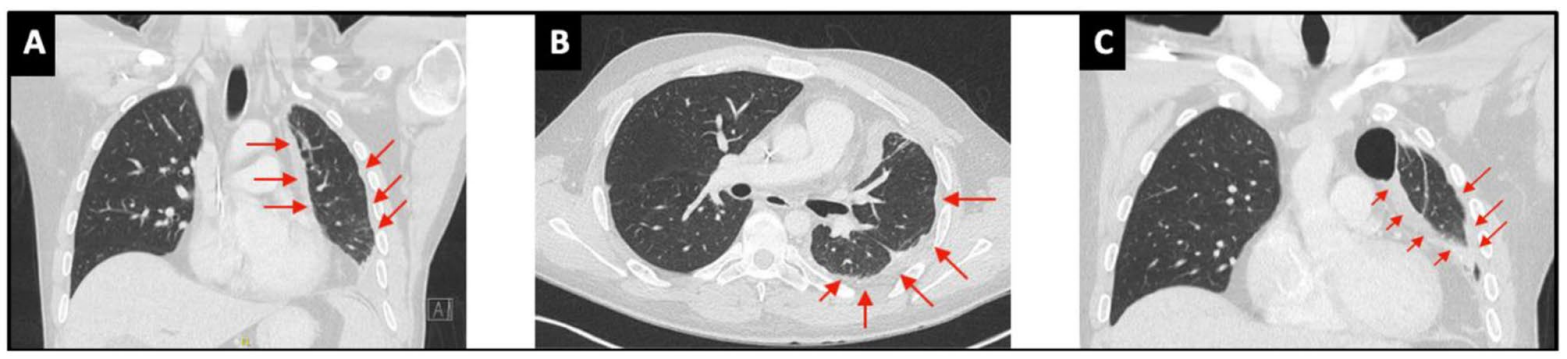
(A, B, C) Chest CT-scan after 6 cycles of neoadjuvant chemotherapy showing stable disease
